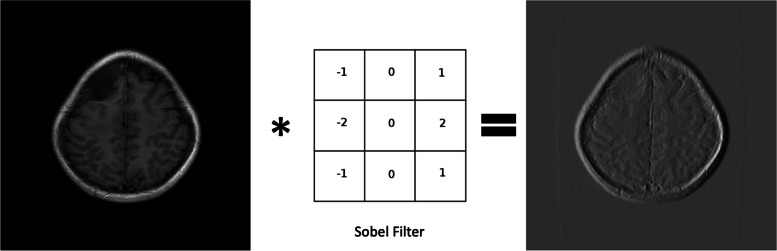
Example of convolutional operation between an input image (a T1-weighted magnetic resonance image of the brain) and the Sobel filter for edge detection
